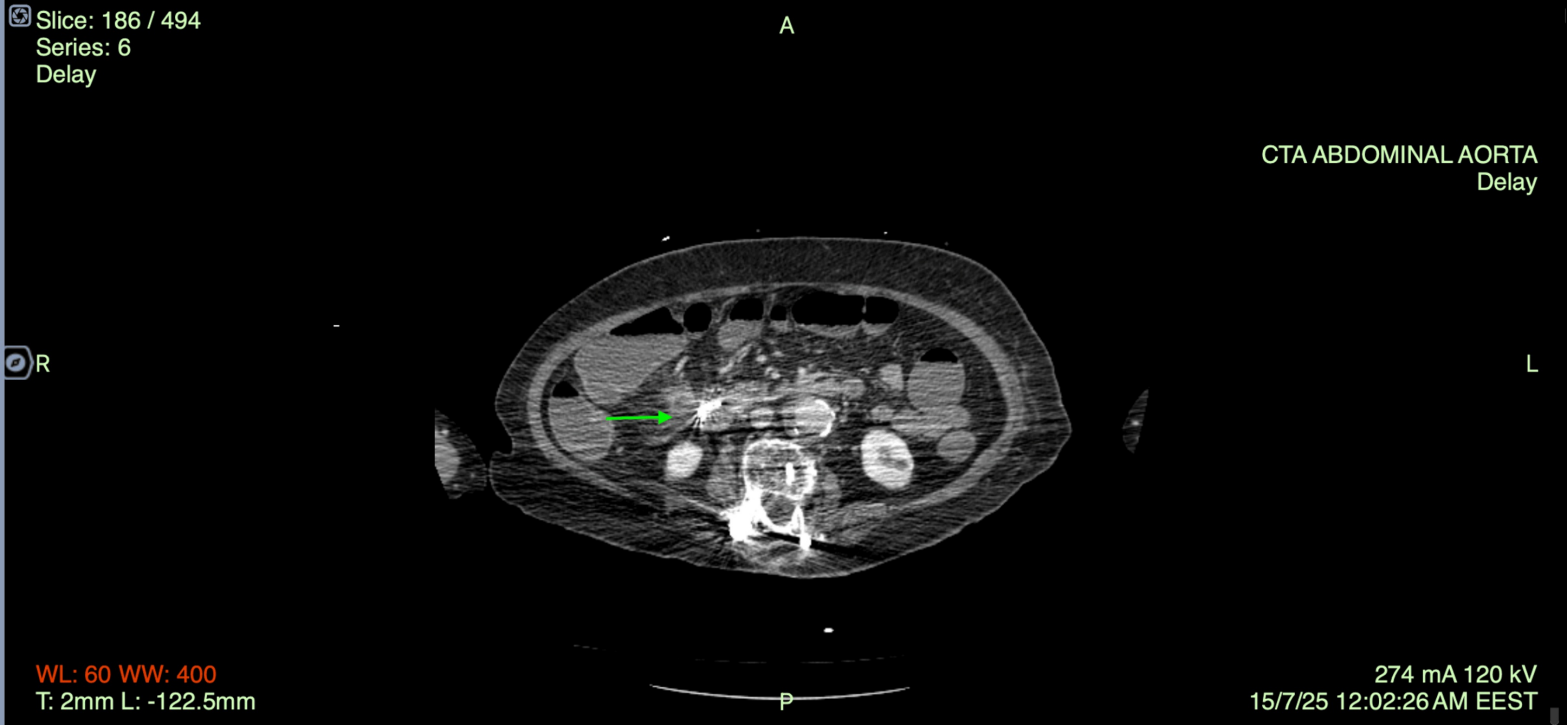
CT angiography - post-clipping.
